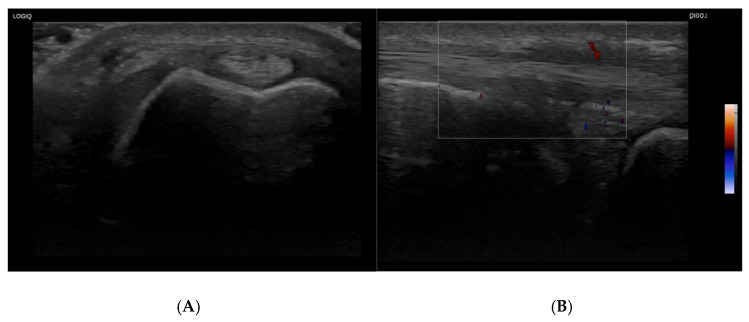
Ultrasound findings of chronic extensor carpi ulnaris (ECU) tenosynovitis. Ultrasound imaging of the extensor carpi ulnaris (ECU) tendon. (A) Transverse view showing synovial sheath thickening consistent with chronic tenosynovitis. (B) Longitudinal view with colour Doppler demonstrating mild ypervascularization, indicative of active inflammatory changes. No evidence of dynamic instability is observed.
